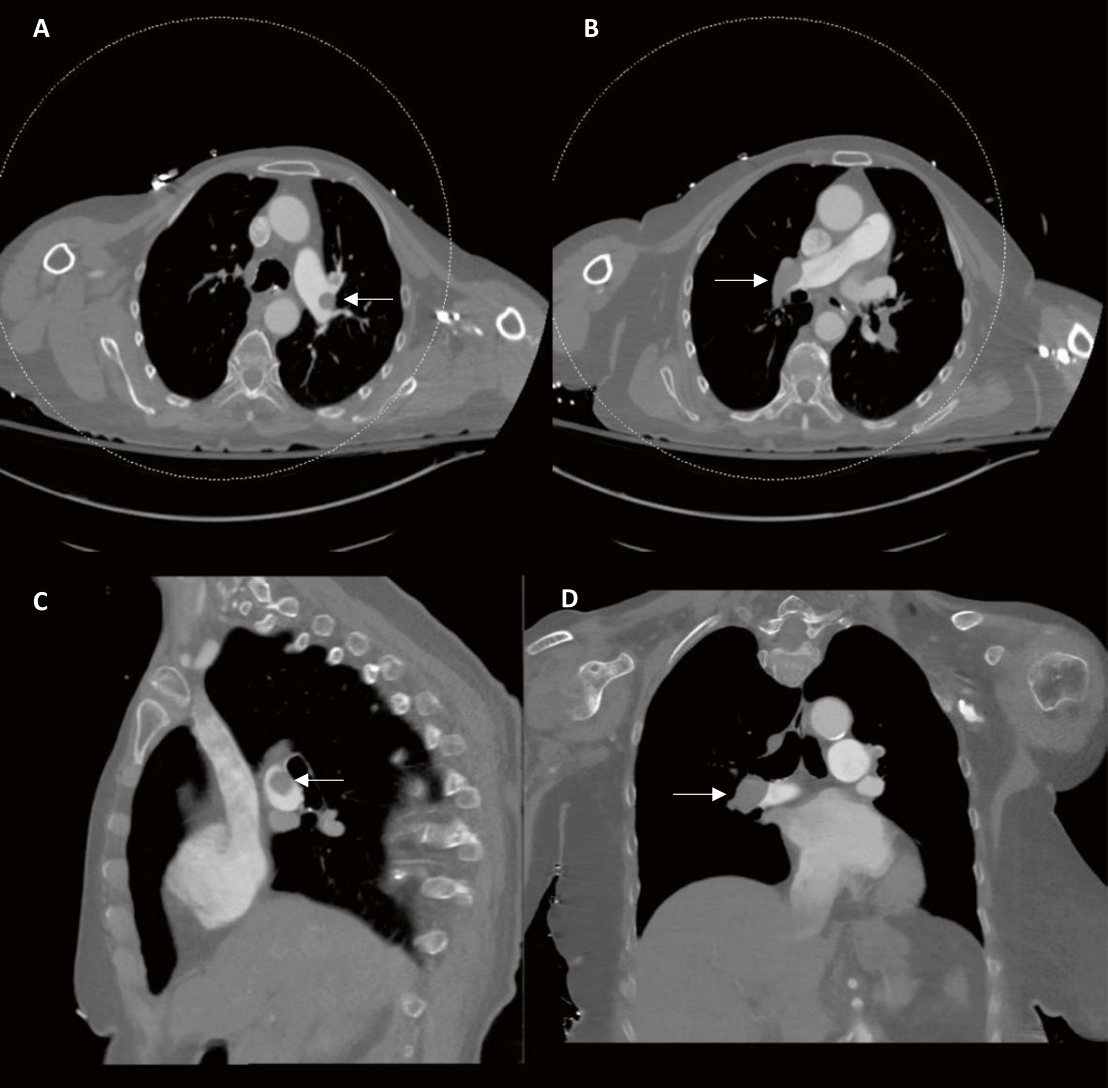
Multiplanar CT images demonstrating bilateral pulmonary arterial filling defects (white arrows)
